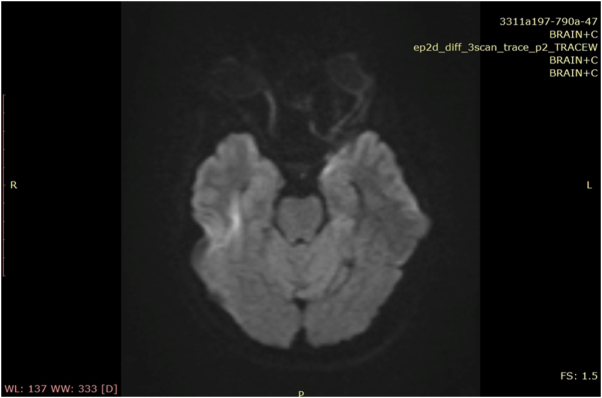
MRI brain showing hyperintensity flair in the right temporal lobe.
